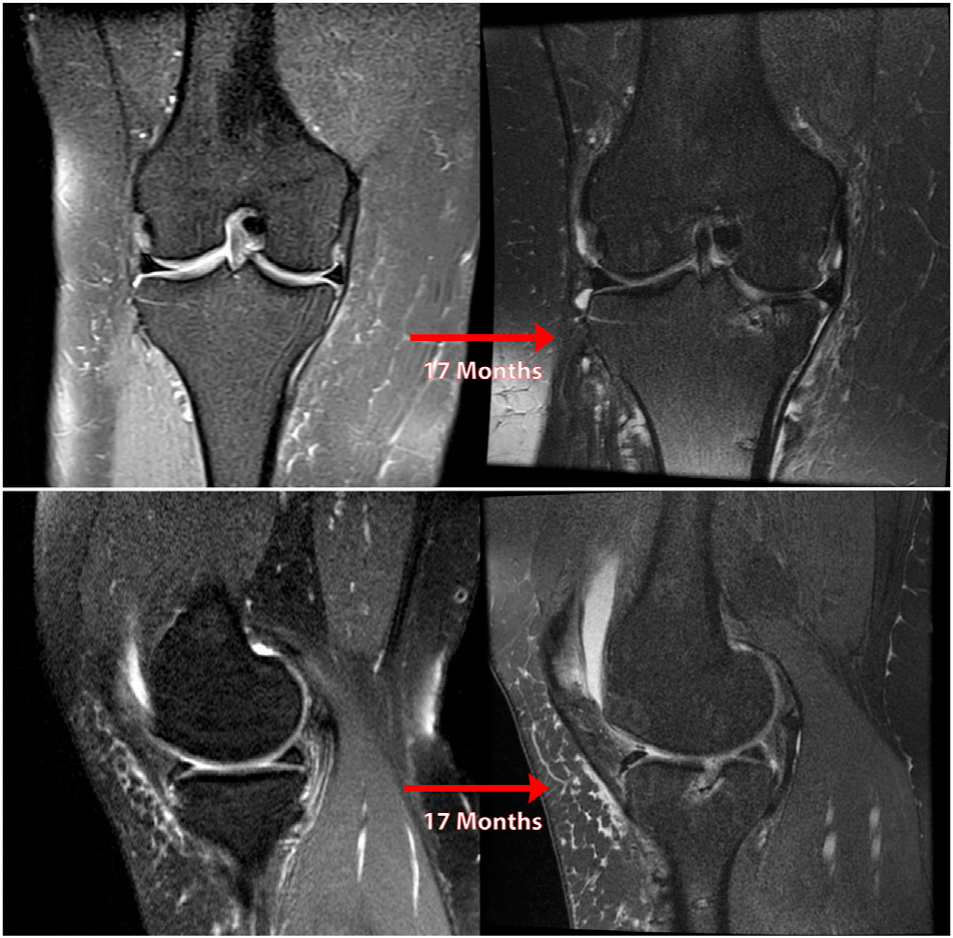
Coronal (A) and sagittal (B) MR images of nonanatomic root repair patient #4 showing progression of osteoarthritis in the medial compartment of the knee over 17 months secondary to nonanatomic medial meniscus root repair (right knee). M = medial; L = lateral; A = anterior; P = Posterior.
